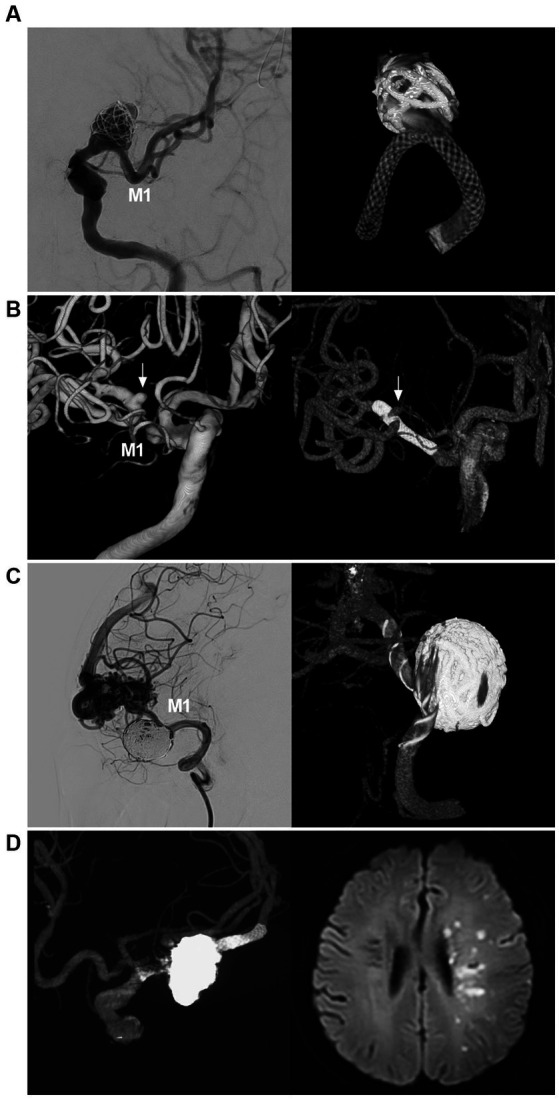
FD deployment in MCA aneurysm. (A) Left panel: DSA showing a wide-necked proximal MCA aneurysm (arrow); right panel: reconstructive CT showing FD deployment in the aneurysm in which the aneurysm was coiled loosely. (B) Left panel: Three-dimensional DSA showing an MCA bifurcation aneurysm (arrow); Right panel: Vaso-3D-DSA showing FD deployment across the bifurcation of the MCA aneurysm (arrow). (C) Left panel: DSA showing that a saccular flow-related aneurysm of the proximal MCA was coiled; right panel: Vaso-3D-DSA showing FD deployment in the aneurysm and that the aneurysm was coiled. (D) Left panel: Vaso-3D-DSA showing that an MCA bifurcation aneurysm was coiled under FD assistance; right panel: postoperative MRI showing acute ischemia of the ipsilateral hemisphere. DSA, digital subtraction angiography; FD, flow diverter, MCA, middle cerebellar artery; M1, first segment of the MCA; MRI, magnetic resonance imaging.
